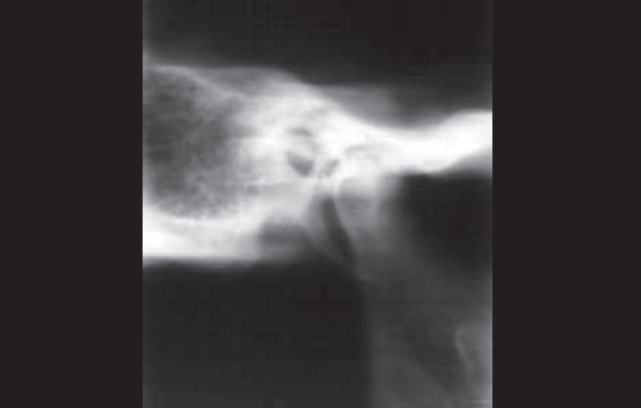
Corrected lateral CTs can - TMJ CT scan sections - section B with barium sulfate.
